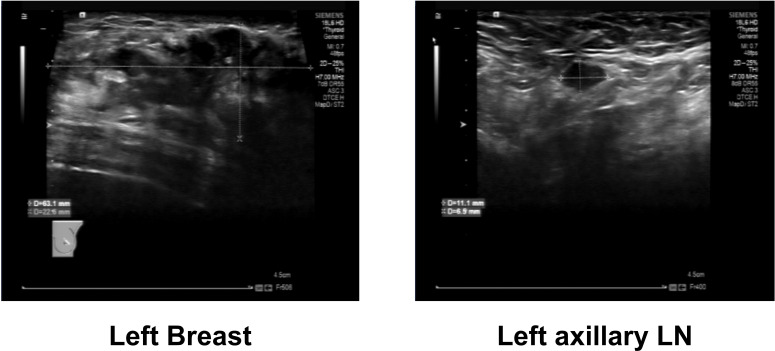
The ultrasound image of the patient's left breast.
